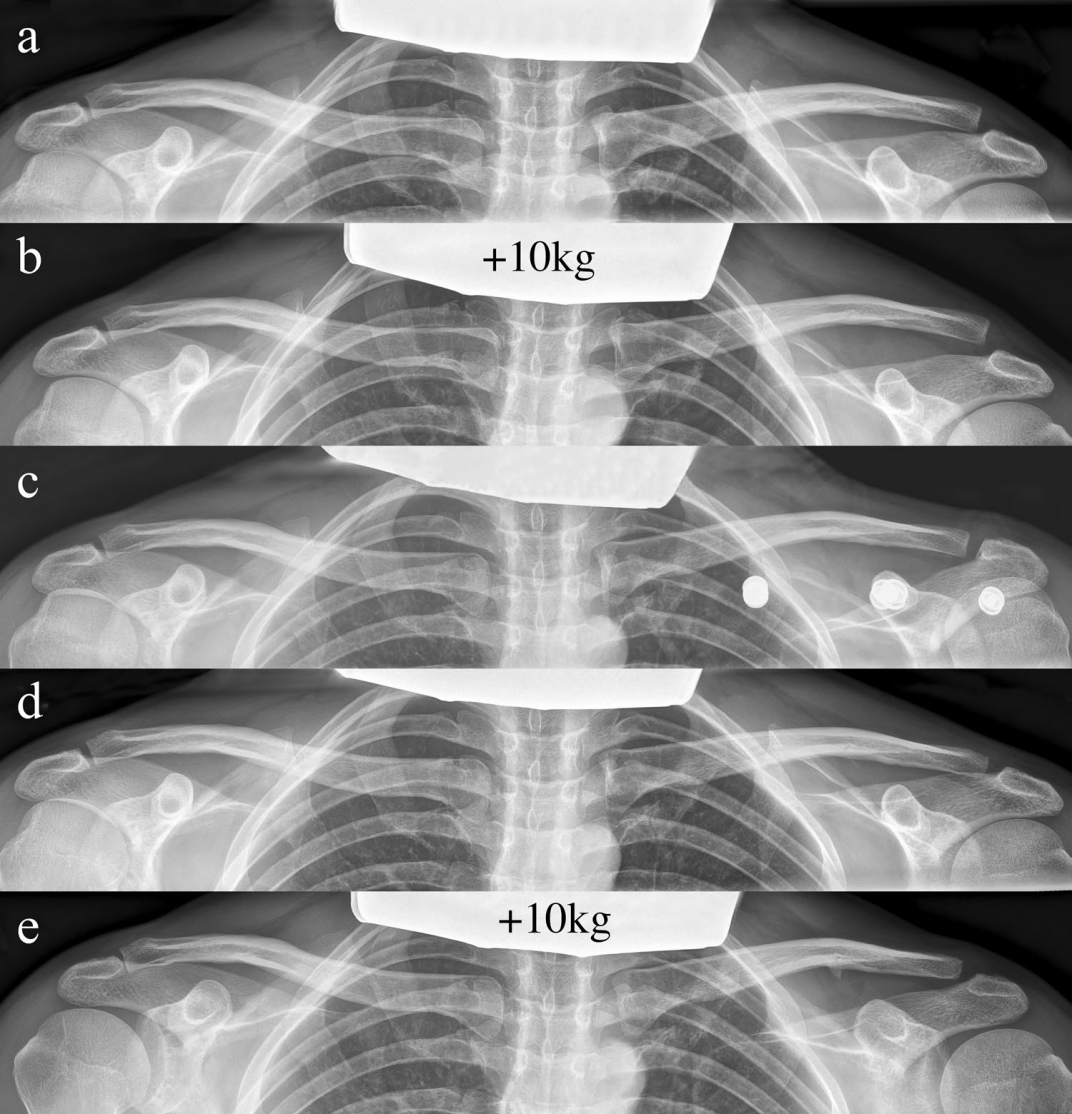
X-ray views at consecutive follow-up appointments. a Unweighted bilateral AP X-ray view of clavicles obtained on the day of injury. A Rockwood type III AC joint separation on the left side is evident. b Weighted bilateral AP X-ray view of clavicles obtained on the day of injury. 10 kg weights were attached to each wrist and unmasked a Rockwood type V AC joint separation with a CC distance of more than 100% compared to the contralateral healthy side (19 mm vs. 8 mm). c Bilateral AP X-ray view of clavicles with the AC joint brace worn on the left side. An anatomic reduction of the left AC joint is visible alongside the projection of three metal buttons just under the left clavicle, stemming from the shoulder pad of the AC joint brace that covers and actively depresses the clavicle. d Unweighted bilateral AP X-ray view of clavicles six weeks after conservative treatment. An anatomic alignment of the left AC joint, comparable to the healthy contralateral side, is evident. e Weighted bilateral AP X-ray view of clavicles obtained at the six-month follow-up. 10 kg weights attached to each wrist show a Rockwood type II AC joint separation with a mildly elevated left clavicle and a small ossification below the middle third of the clavicle
